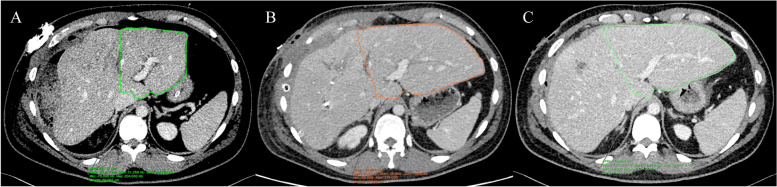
CT-scans that demonstrate regeneration of non-damaged left lobe of liver
